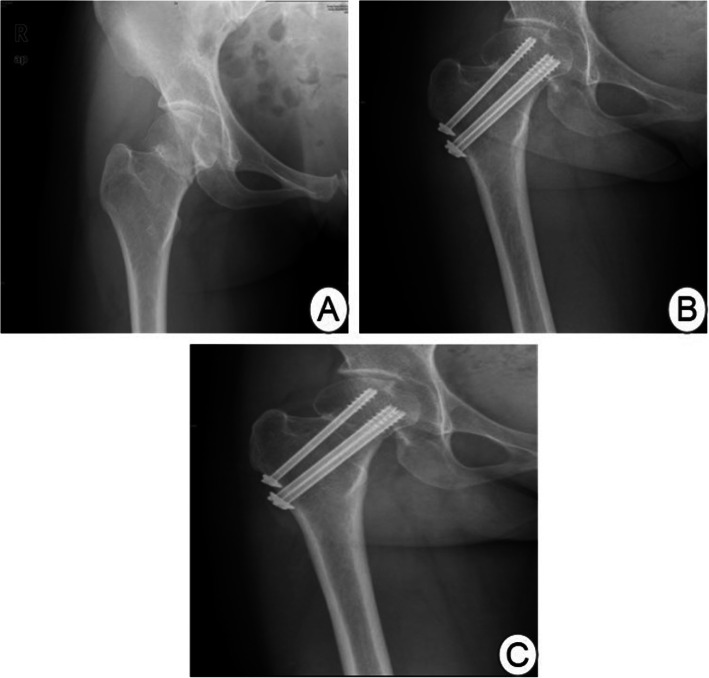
a Preoperative X-ray film. b At 1 year after the operation, X-ray examination showed that the necrosis of the right femoral head. c At 2 years after the operation
